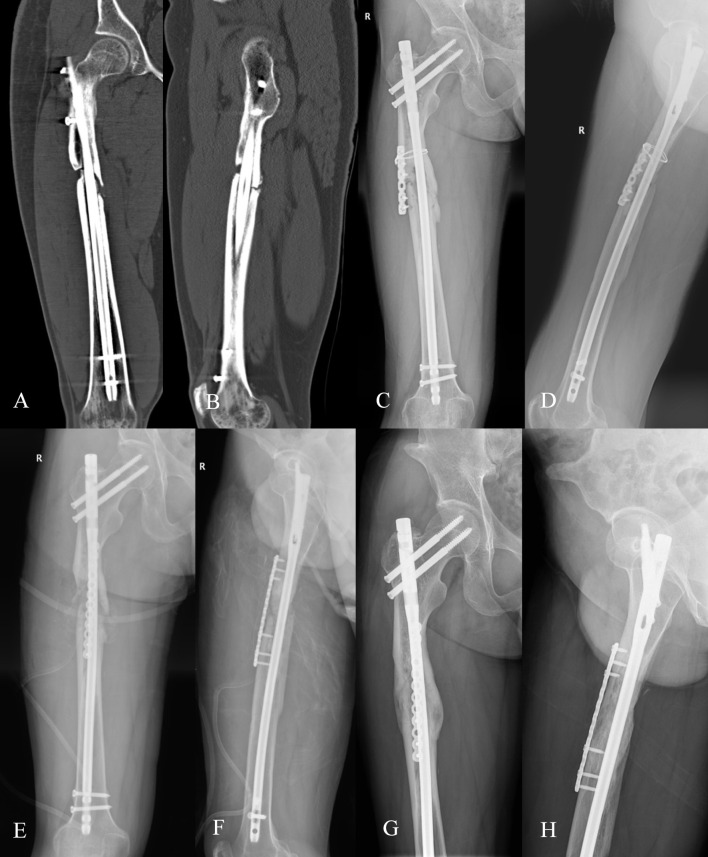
A 51-year-old male patient with a body mass index of 25.60 kg/m^2^ and a 15-year smoking history. In September 2020, he sustained a right femoral shaft fracture due to a traffic accident and underwent closed reduction and intramedullary nailing at another hospital; a re-examination 10 months postoperatively revealed fracture nonunion, and he underwent the first revision surgery (nail exchange + auxiliary plate internal fixation + autologous iliac bone grafting) at the original hospital in August 2021; a re-examination in December 2022 still showed fracture nonunion, and he was subsequently referred to our hospital. (A,B) X-ray films before the first revision surgery (9 August 2021), showing right femoral shaft fracture nonunion with bone resorption at the fracture end; (C,D) X-ray films 16 months after the first revision surgery (15 December 2022), showing persistent fracture nonunion and backout of the distal screws of the auxiliary plate; (E,F) X-ray films after the second revision surgery (22 December 2022), surgical method: auxiliary plate exchange + platelet-rich plasma (PRP) combined with autologous iliac bone grafting; (G,H) Outpatient follow-up X-ray films 10 months postoperatively (19 October 2023), showing satisfactory fracture healing; functional scores: SF-36 score 81 points, Harris Hip Score 91 points, Visual Analog Scale score 0 points.
